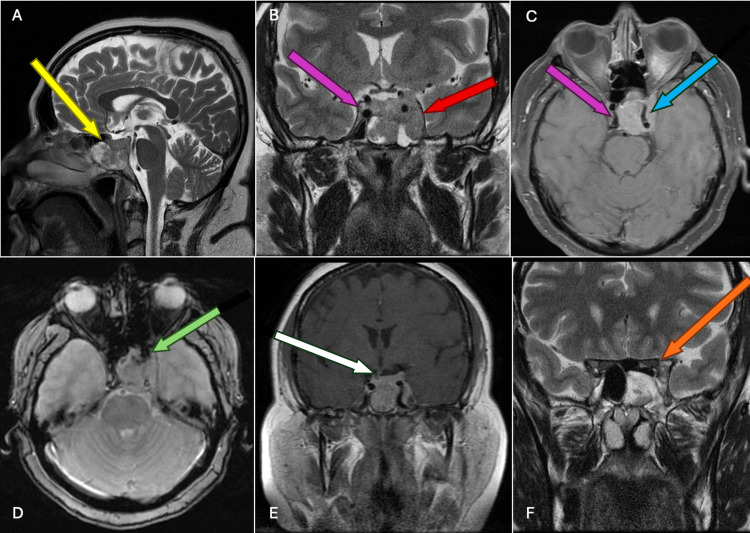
(A) Sagittal T2-weighted MRI of the brain demonstrates a well-defined, heterogeneous lesion occupying the sella turcica with inferior extension into the left sphenoid sinus (yellow arrow). (B) Coronal T2-weighted image shows extension into the left cavernous sinus with early superior extension into the middle cranial fossa (red arrow) and medial abutment of the right ICA (purple arrow). (C) Axial T1 post-contrast image demonstrates encasement of the left ICA (blue arrow) and medial abutment of the right ICA (purple arrow). (D) Axial T2-weighted GRE image demonstrates susceptibility artefact within the lesion, in keeping with haemorrhagic components suggestive of pituitary apoplexy (green arrow). (E) Coronal T1 post-contrast image demonstrates rightward deviation of the pituitary stalk (white arrow), which is continuous with the lesion and undifferentiable. (F) Coronal T2-weighted image demonstrates encasement of the ipsilateral optic nerve (orange arrow). MRI: magnetic resonance imaging; GRE: gradient recalled echo; ICA: internal carotid artery
